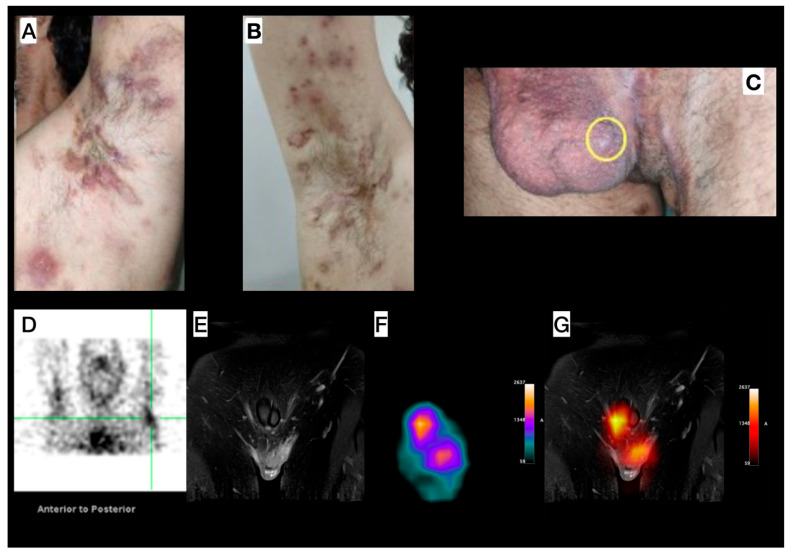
Patient 3—Left (A) and right (B) axillary region images in different disease stages, concomitantly (comedones, abscesses, inactive lesions, and scars). (C) Image of the genital and left inguinal region; scrotum presenting an inflammatory nodule (yellow circle). (D) SPECT image acquired from the head to the thoracic region, with the upper limbs elevated, showing abnormal uptake in both the right and left axillary (green reticle) regions. (E–G) Pelvic images: Magnetic Resonance Imaging (MRI) (E) shows focal scrotal wall thickening characterized by increased signal intensity on Short Tau Inversion Recovery (STIR) sequences and areas of enhanced contrast uptake, which are more prominent on the left side and extend into the inguinal regions. SPECT image (F) shows abnormal radiotracer uptake within the scrotum. (G) MRI and SPECT findings, enabling precise anatomical localization of the SPECT uptake.
